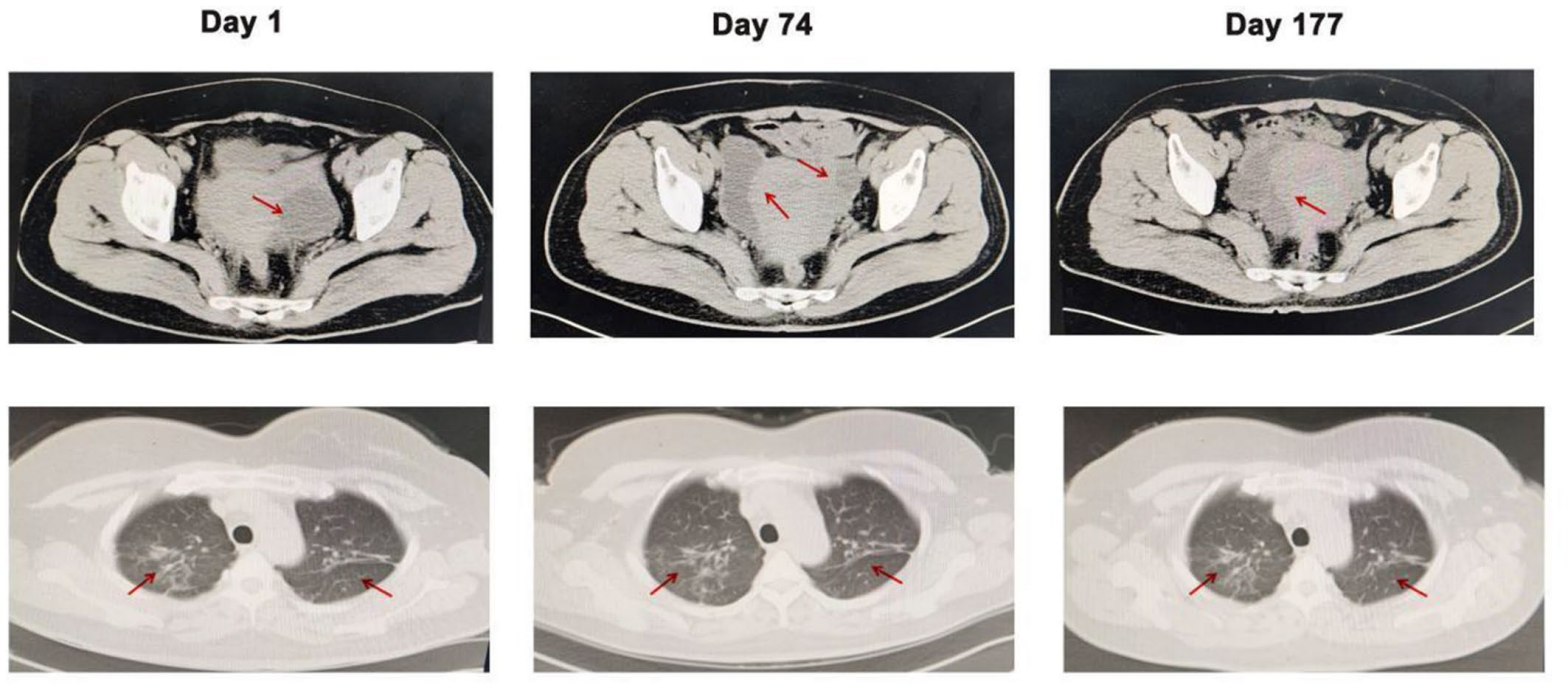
Computed tomography of the abdomen and chest on days 1, 74, and 177. Arrows indicate the lesions in the abdomen and lungs. Compared with the CT results on days 1 and 177, the low-density cystic lesion in the left adnexal area cavity disappeared, but patchy high-density lesions in both lungs did not improve.
